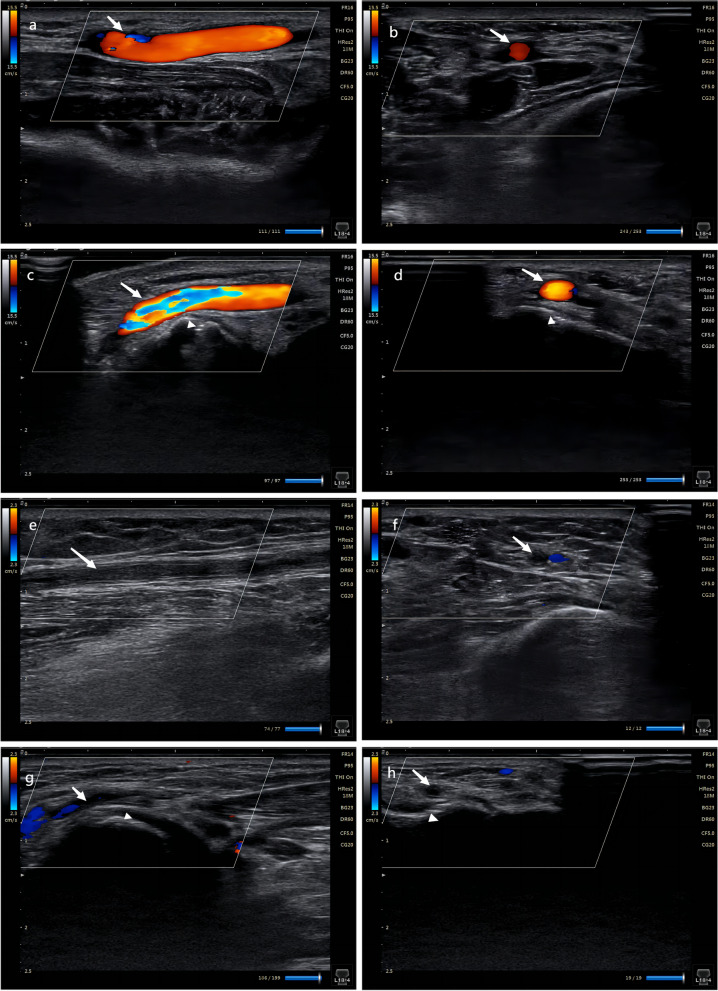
Color Doppler images of the proximal RA and the distal RA. Long axis (a) and short axis (b) images of a normal proximal RA (white arrow). Long axis (c) and short axis (d) images of a normal distal RA (white arrow). Long axis (e) and short axis (f) images of an occluded proximal RA (white arrow). Long axis (g) and short axis (h) images of an occluded distal RA (white arrow). The distal RA lies on the dorsal surface of the scaphoid (arrowhead). RA, radial artery
